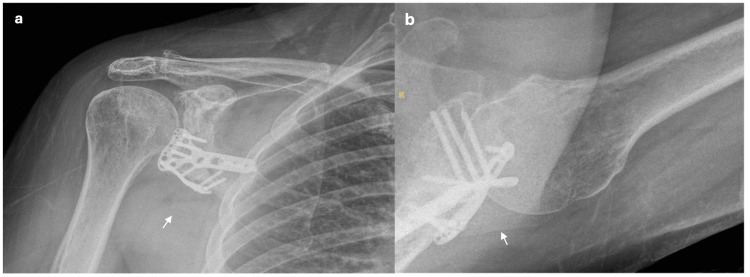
Seven-month post-operative shoulder radiographs. a. Anterior-posterior right shoulder X-ray. b. Axial right shoulder radiograph. Figures 5a-5b show a well-healed fracture with the maintained position of the distal radius plate seven months post-operatively (as indicated by arrows).
